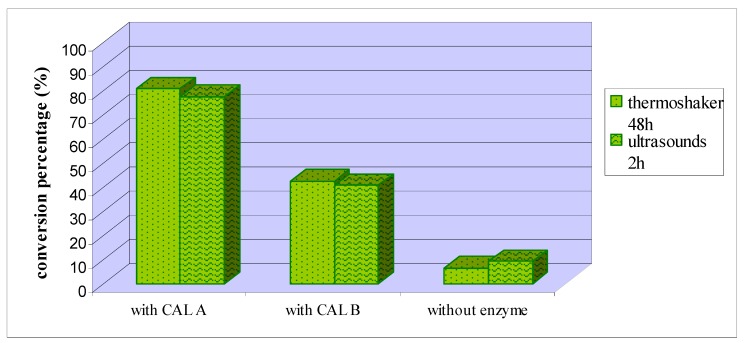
Conversion percentage to indolizine 1a in enzymatic and non-enzymatic *one-pot* synthesis, carried out with and without ultrasounds.
